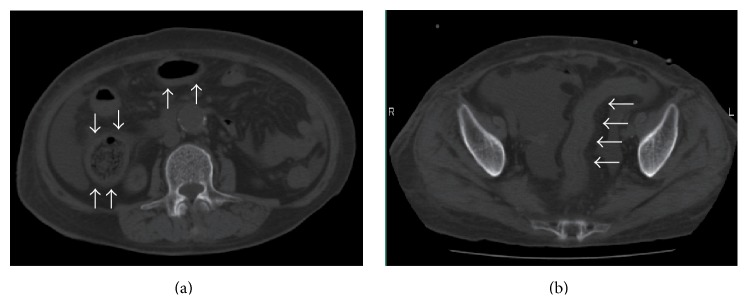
Computed tomography before treatment. Diffuse wall thickness of (a) the ascending colon and transverse colon and (b) the sigmoid colon (white arrows).
